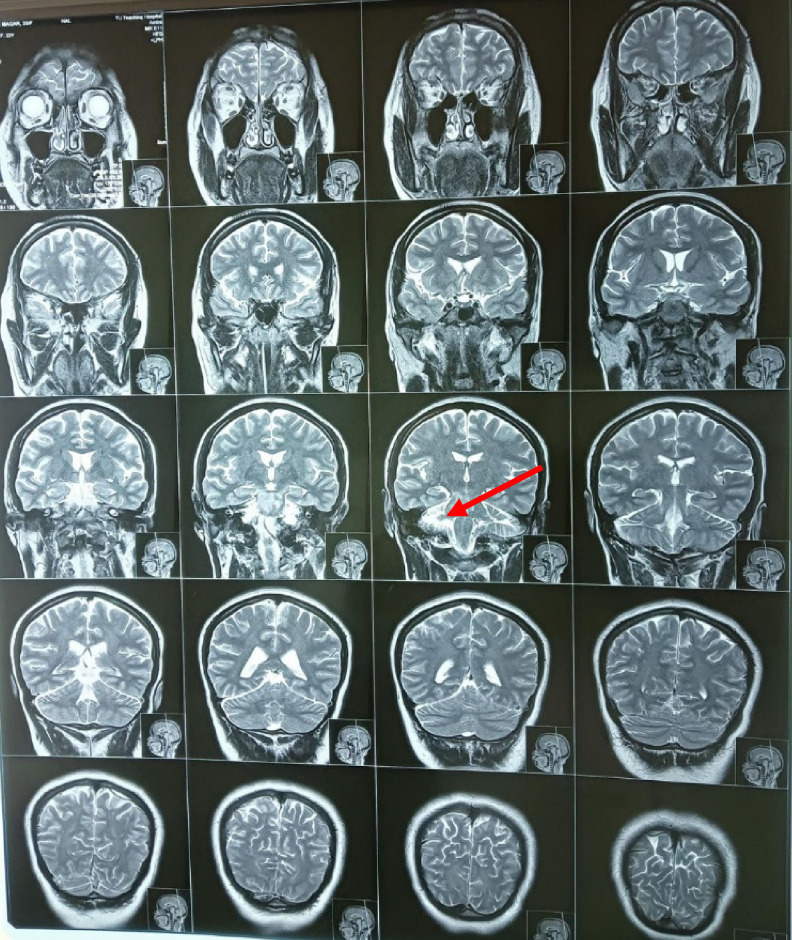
MRI study: There is a focal area of T2/FLAIR high signal intensity noted in the right cerebellar hemisphere.
